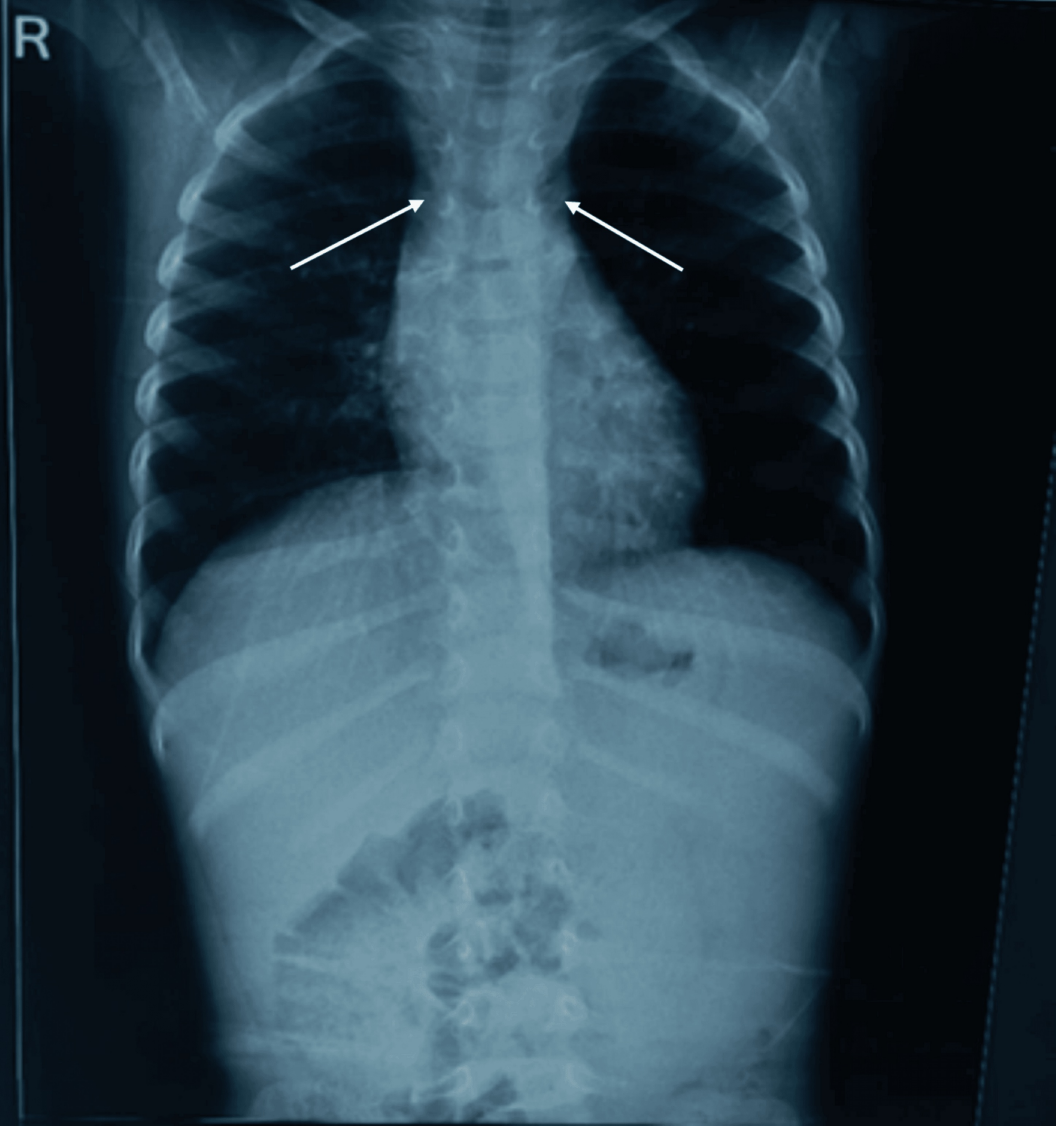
Chest X-ray reveals mediastinal lymphadenopathy with normal lung parenchyma (indicated by arrows)
